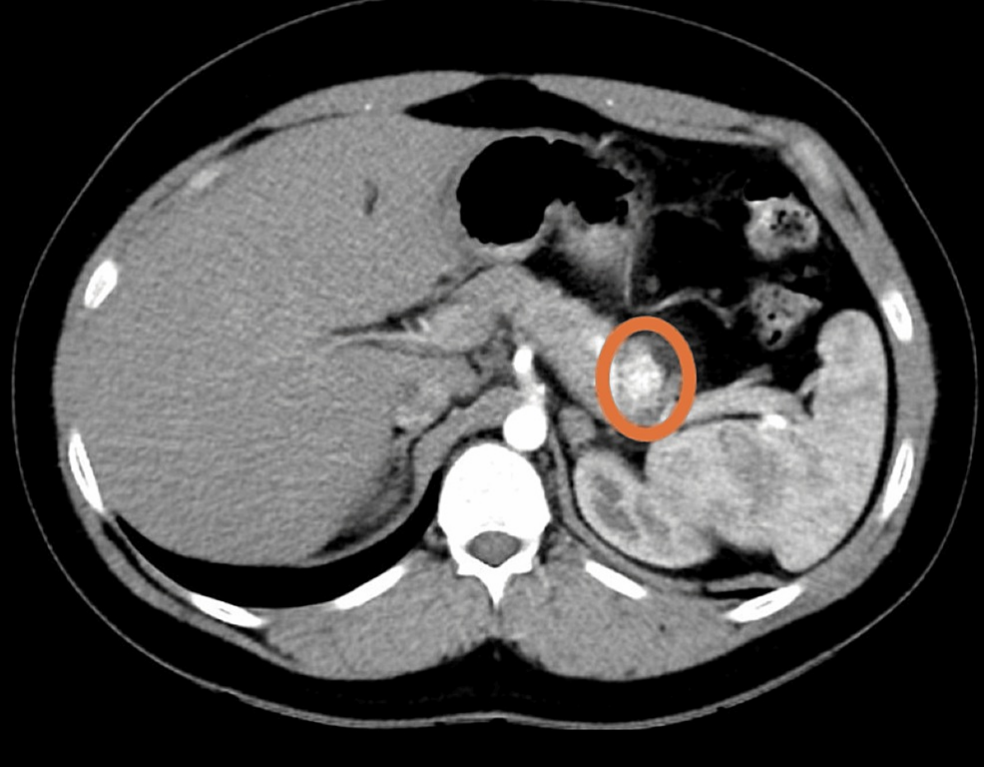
Abdominal computed tomography revealing well-defined hyperdense lesion in the body pancreas.
